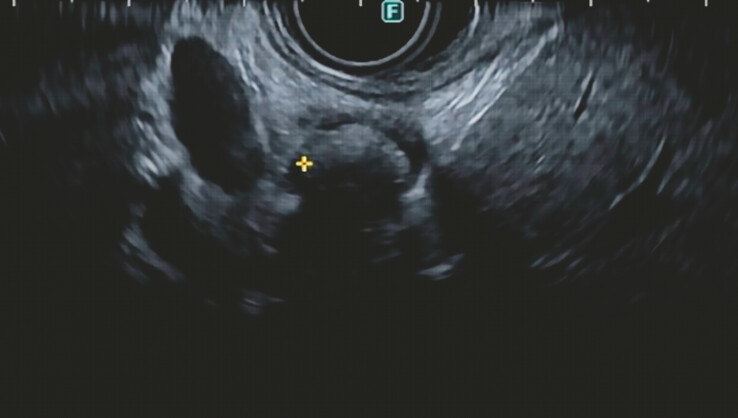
EUS image showing a 9-mm stone lodged in the common hepatic duct near the cystic duct take-off. Abbreviation: EUS, endoscopic ultrasound.
